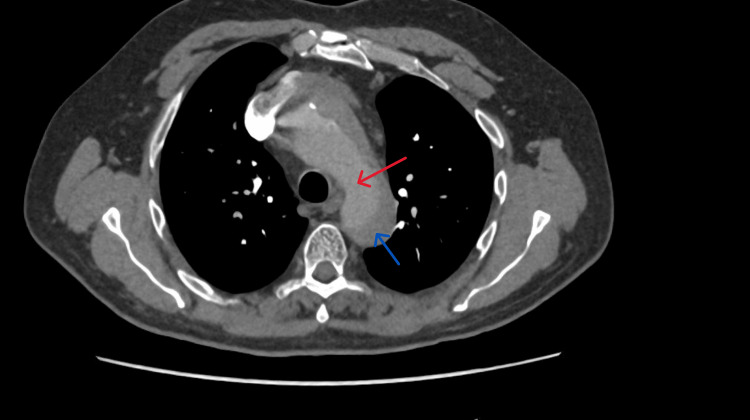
CTPA in axial view. Computed tomography pulmonary angiogram (CTPA) in axial view showing the dissected aortic arch. There is no contrast opacifying the aortic arch because this computed tomography (CT) scan was performed as a dedicated pulmonary embolism study, with contrast timing optimised for the pulmonary arterial circulation. Red arrow: true lumen; Blue arrow: false lumen.
